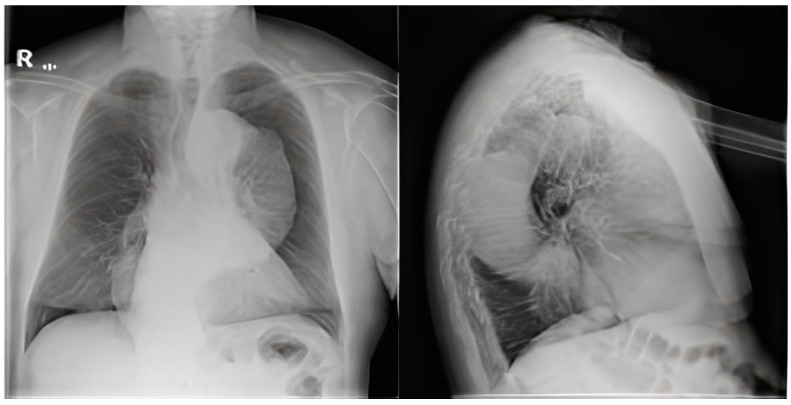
Chest radiograph of a patient presenting to the emergency department with dyspnea. The AP and LL projections reveal an incidentally detected thoracic aortic aneurysm, showing marked ectasia of the aortic arch with contralateral tracheal displacement.
